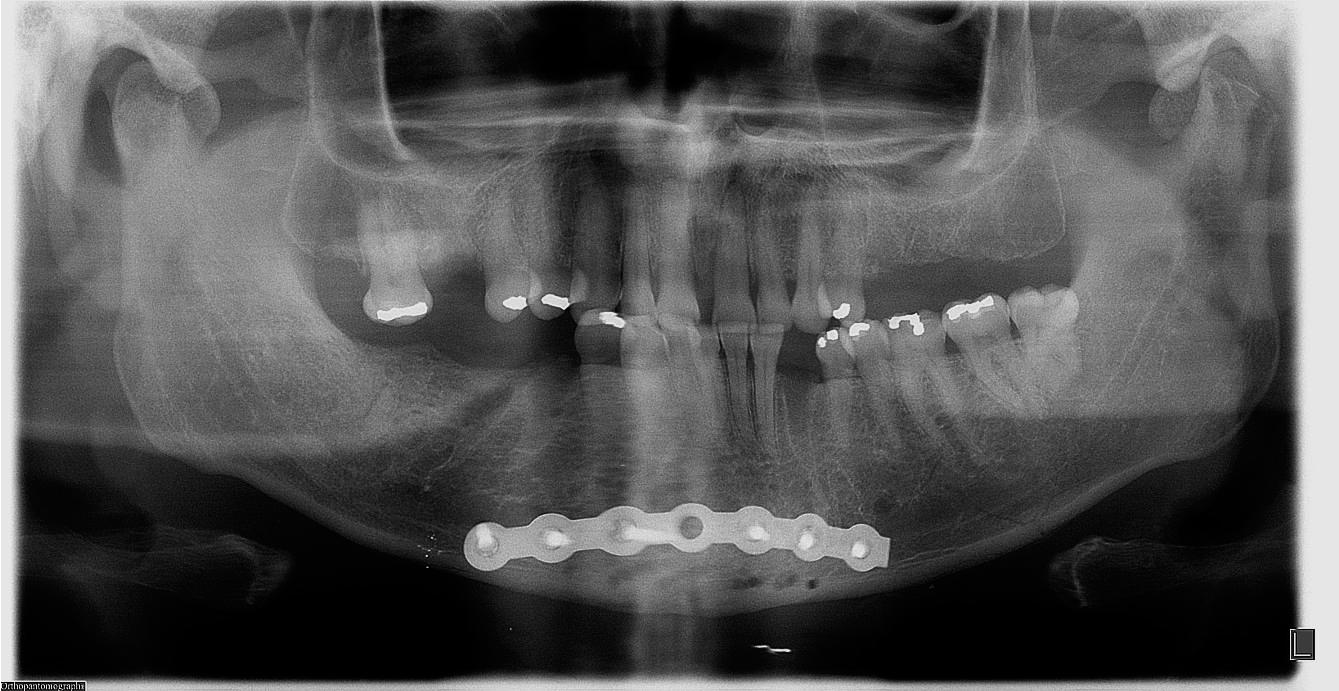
Ortopantomogram radiography showing internal rigid fixation with 2.4 mm reconstruction plate.
